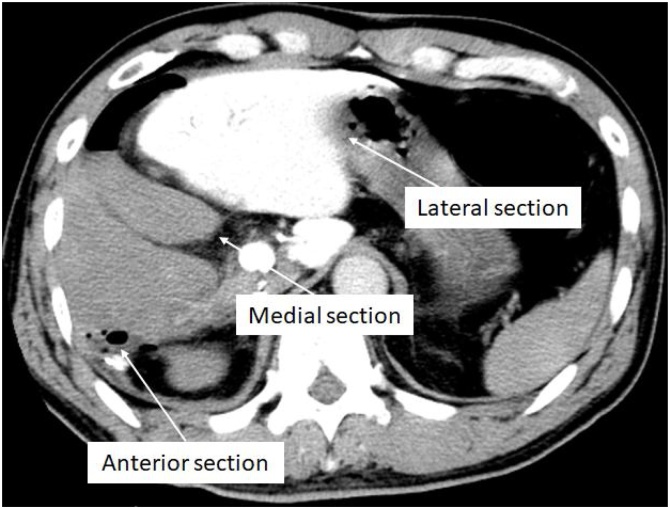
Only the lateral section is enhanced. The medial and anterior sections are not enhanced on computed tomography during arterial portography. The remnant liver migrates into the right subphrenic space.
